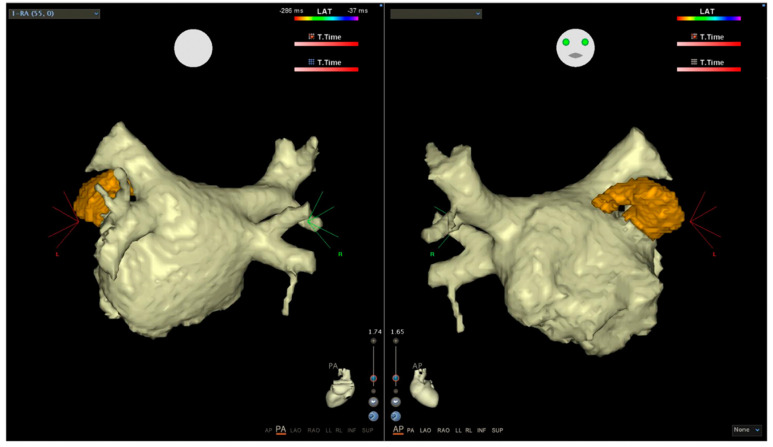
Reconstruction of the LA, pulmonary veins, and left appendage with the true anatomy using multislice computed tomography.
